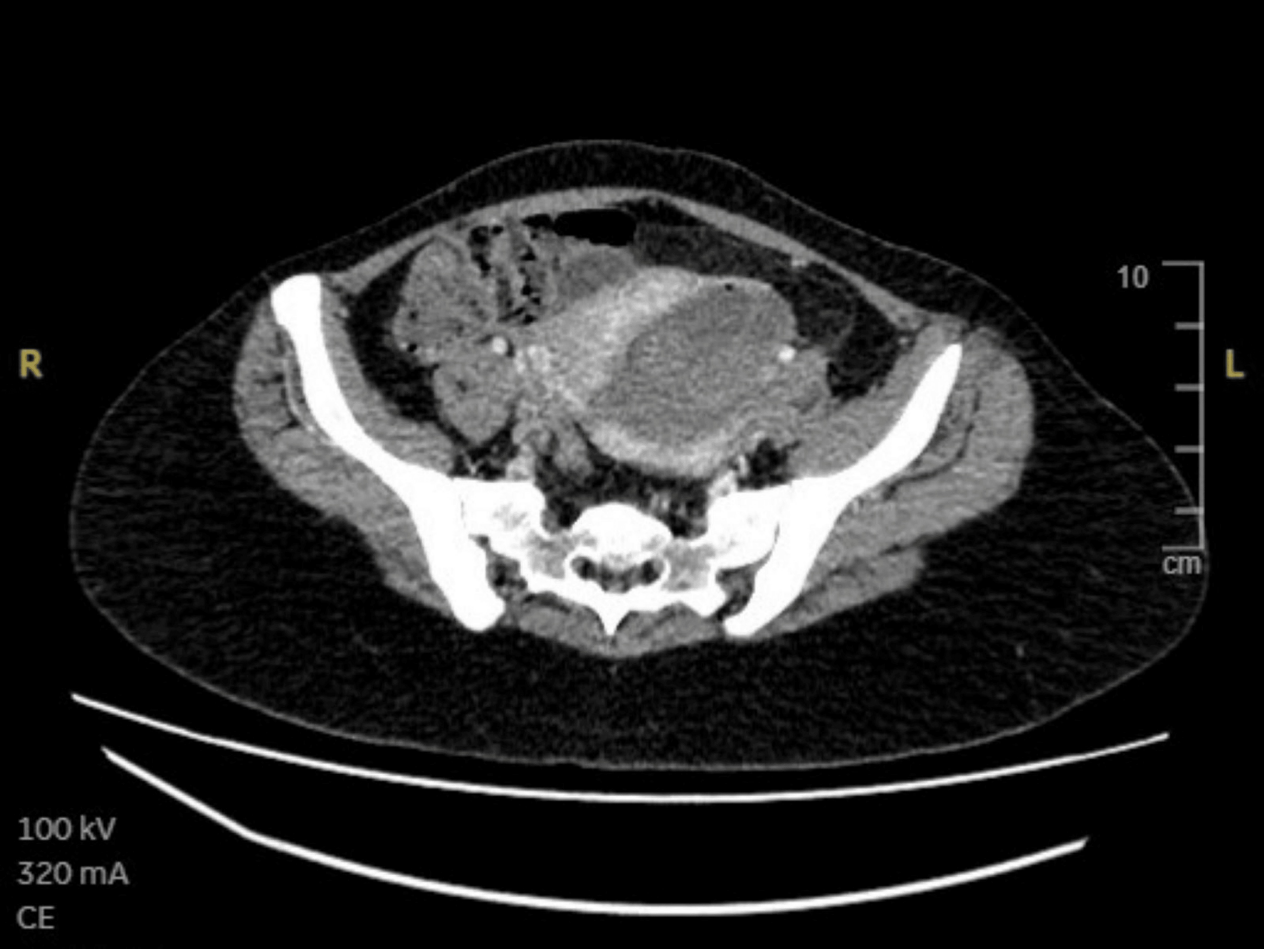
CT scan, which was inconclusive for uterine wall compromise.
